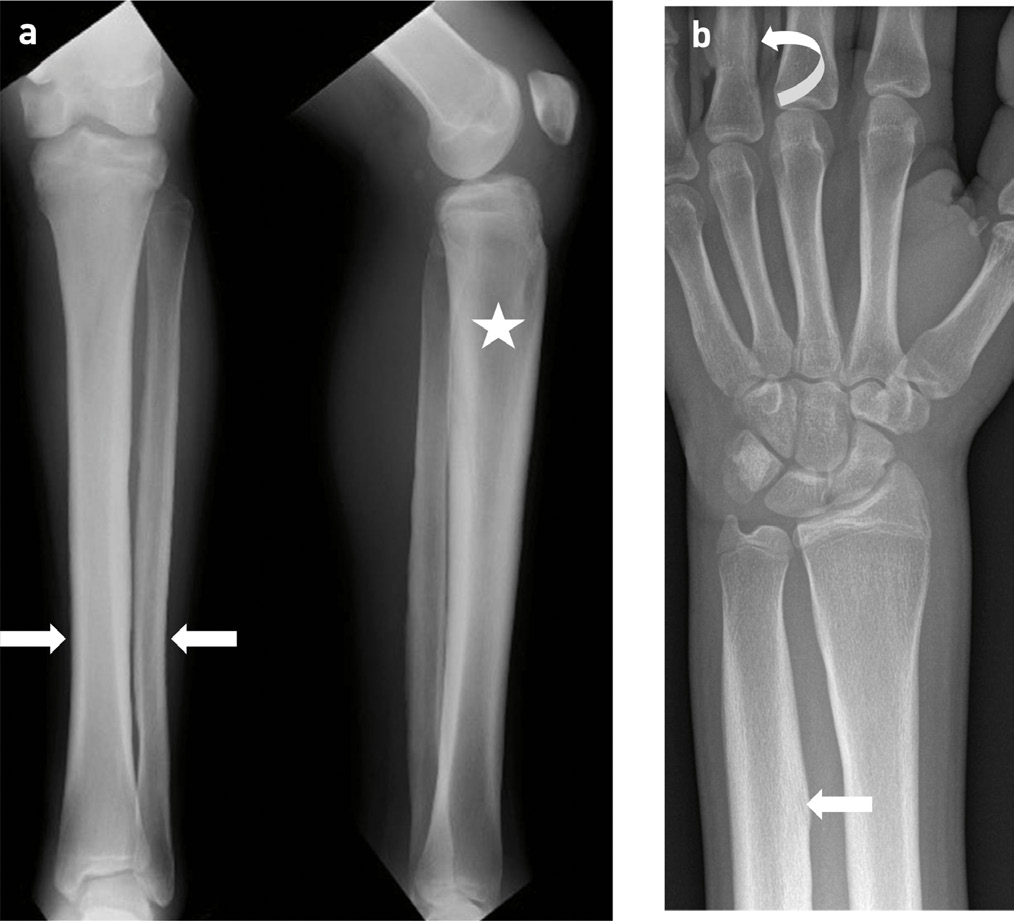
12-year-old boy with primary hypertrophic osteoarthropathy. (a) AP and lateral radiographs of the left lower leg demonstrating diffuse lamellar periosteal reaction and cortical thickening of the tibia and fibula (arrows) with enlargement of the bone (star). The appearances of the contralateral leg were identical (not shown). (b) AP radiograph of the right wrist demonstrating diffuse cortical thickening of the radius and ulna (arrow) with enlargement of the bones. Further cortical thickening in the proximal phalanges of the fingers (curved arrow) is seen. The changes were symmetrical with identical appearances in the contralateral upper limb (not shown).
